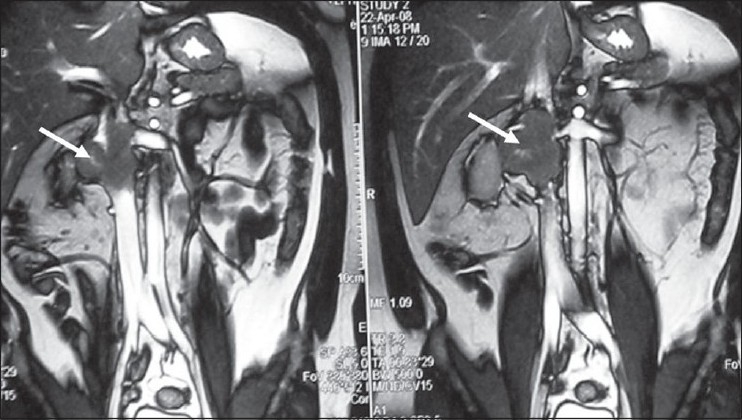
MRI TRUFISP coronal images showing IVC tumor (arrow) extending into right vein and infi ltrating right renal hilum. The left renal vein junction with IVC is also involved by the tumor
